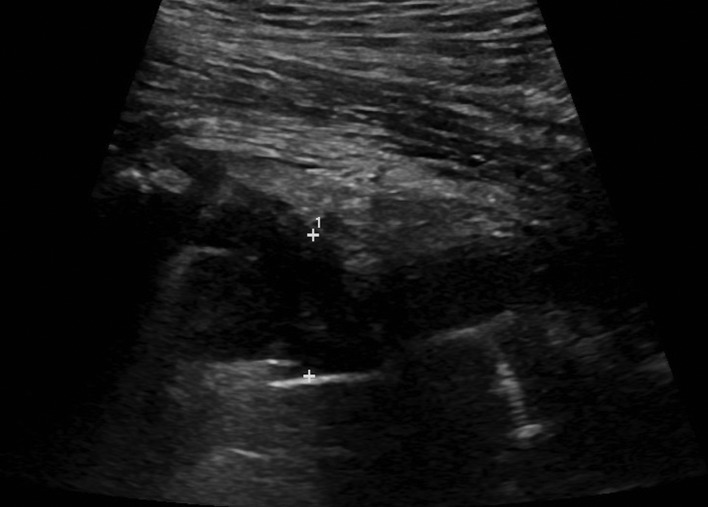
Ultrasound scan of the right hip showing a hip joint collection with a depth of 18 mm.
